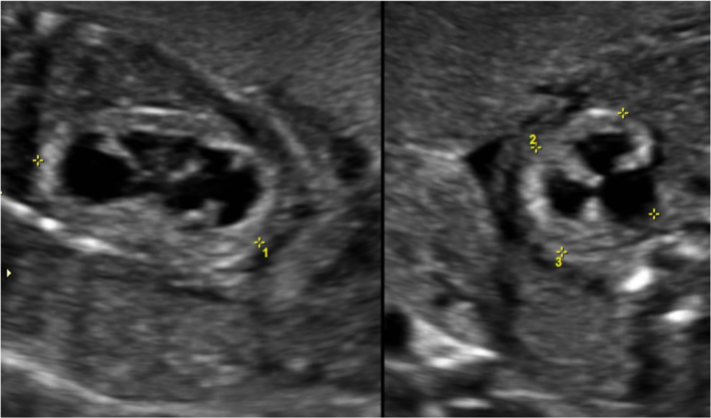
Ultrasound image of hyperechoic and small for gestational age fetal kidneys, secondary to fetal lower urinary tract obstruction
